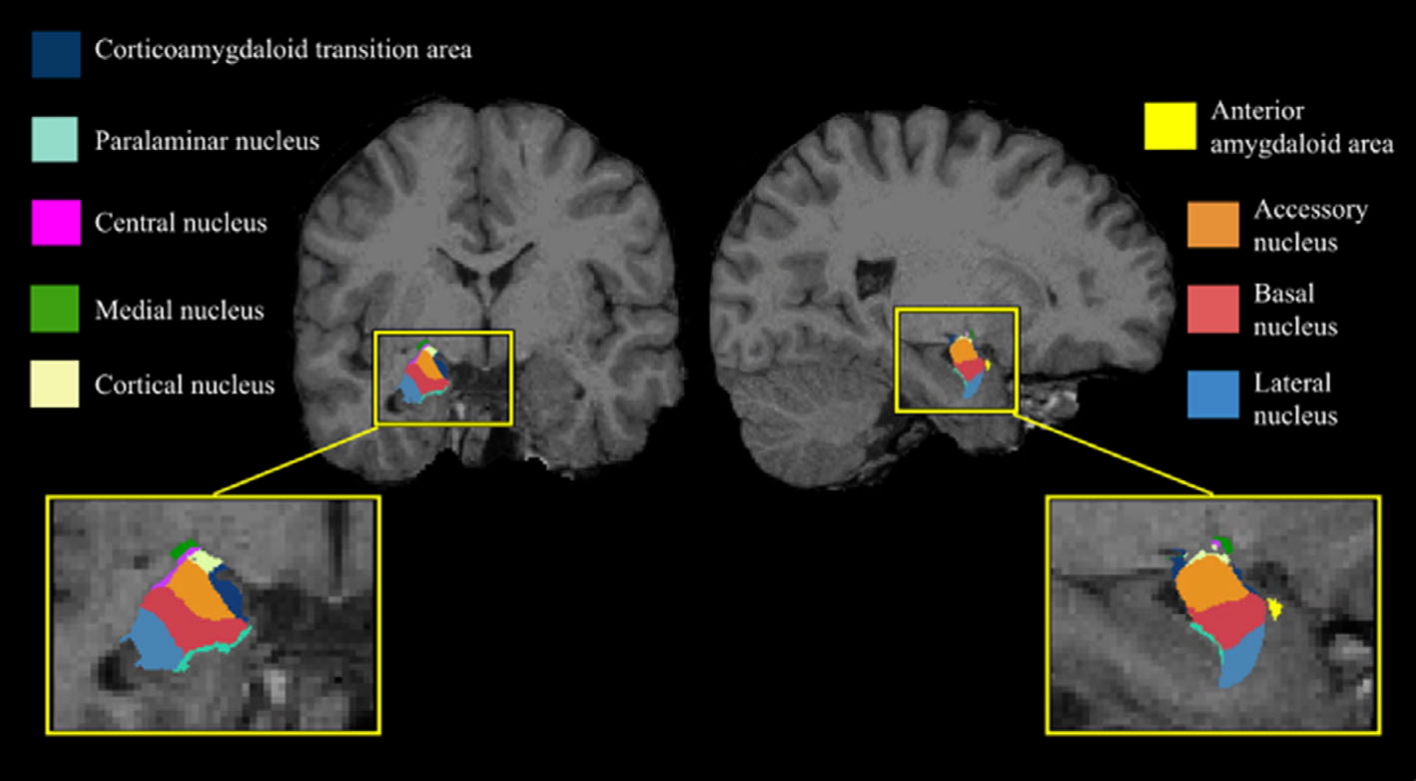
Amygdala atlas registered to a representative T1‐weighted image obtained in a healthy control participant. CTA, corticoamygdaloid transition area
